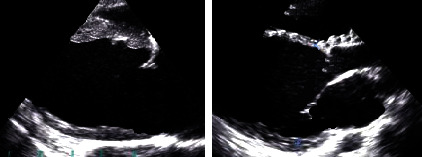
Echocardiograms of a patient with heart failure. Female, 63 years old.
